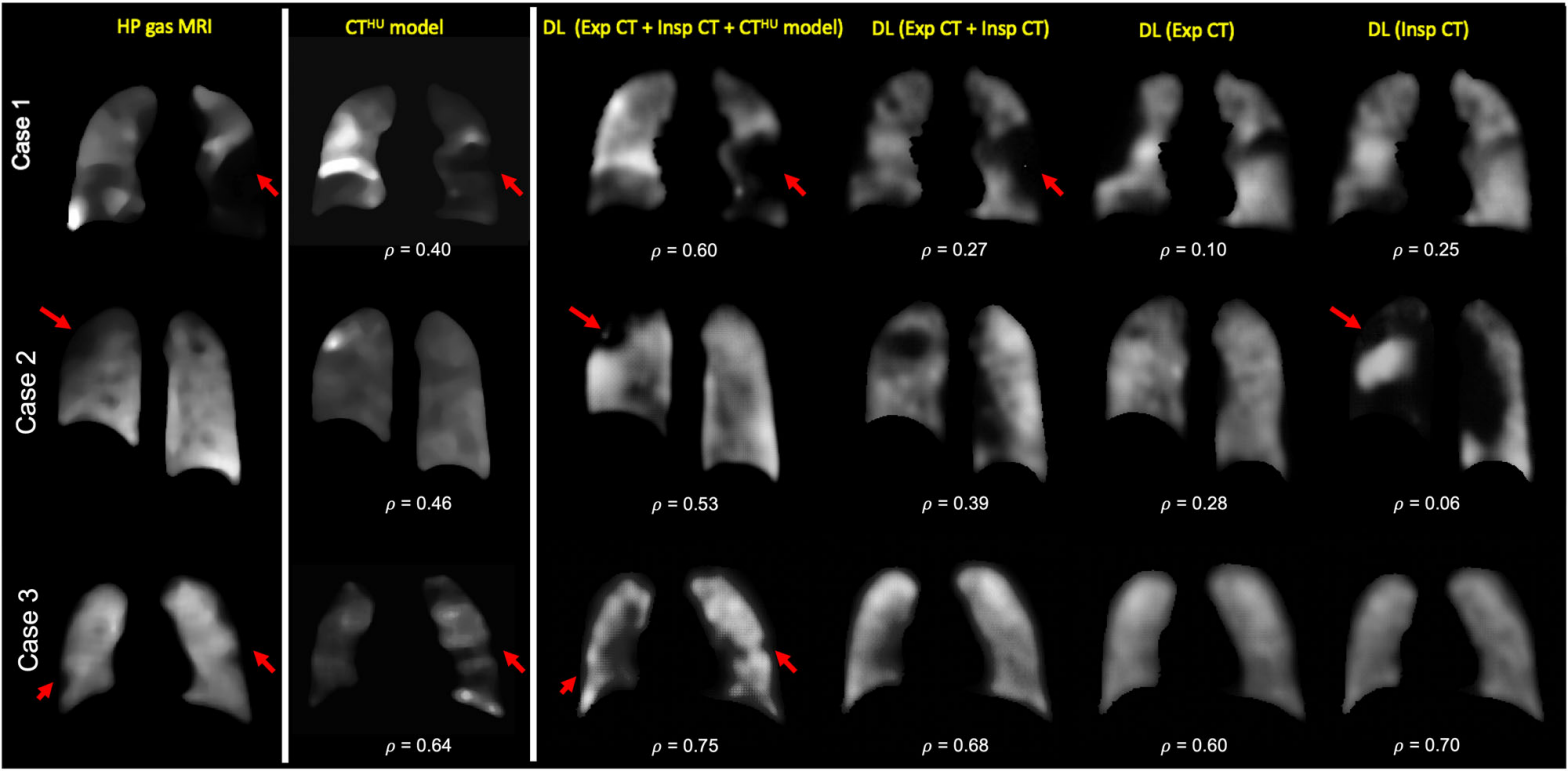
Example coronal slices from the CT^HU^ model and the four DL frameworks for three cases compared to ^3^He MRI. Spearman's 𝜌 values between each method and ^3^He MRI are provided. Red arrows demonstrate examples of replicated defects. Cases 1 and 2 are from lung cancer patients and Case 3 is from a cystic fibrosis patient.
